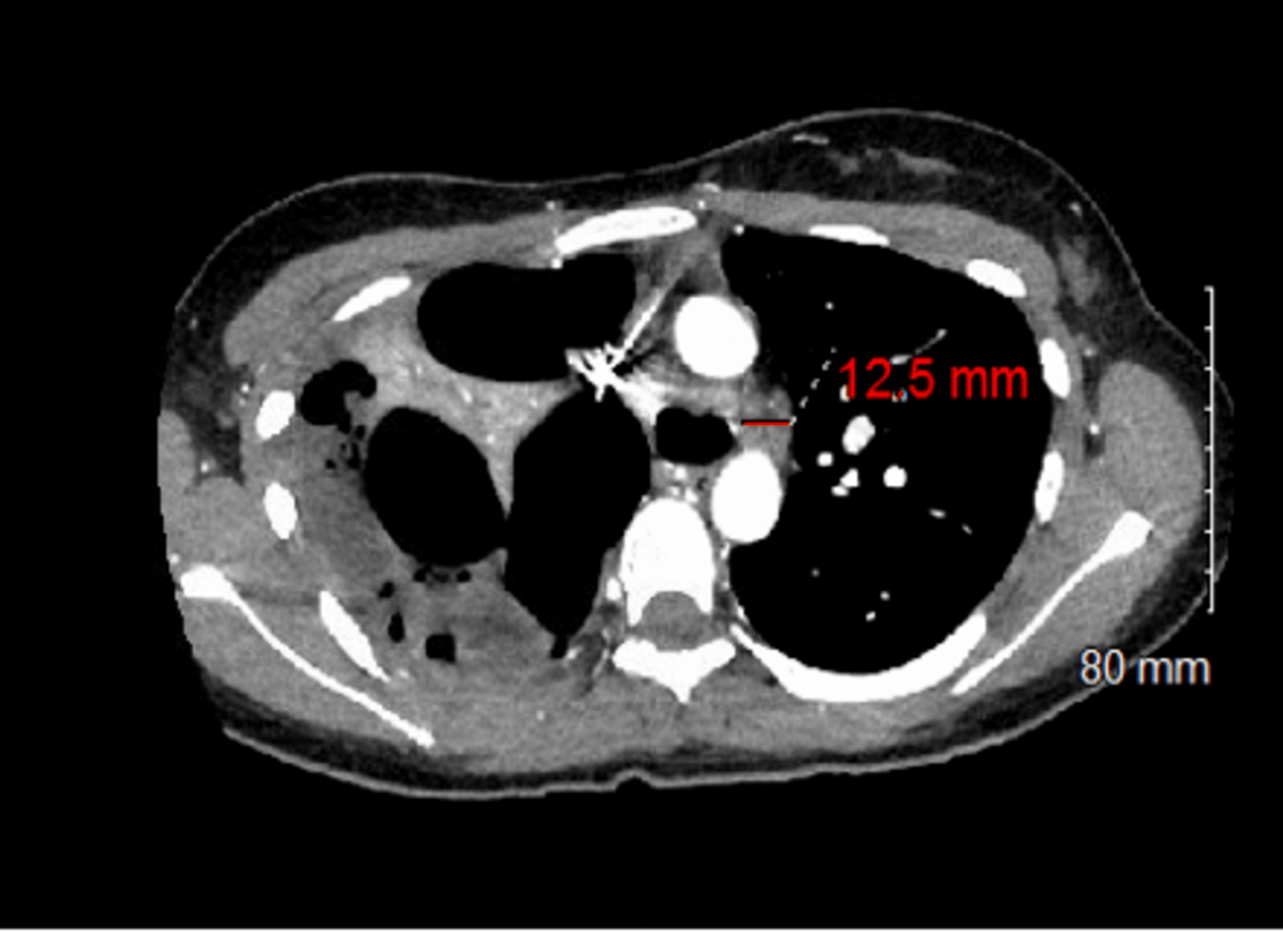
CT scan demonstrating occlusion of the right mainstem bronchus, right lung collapse, and multiloculated pleural collection with gas bubbles.
